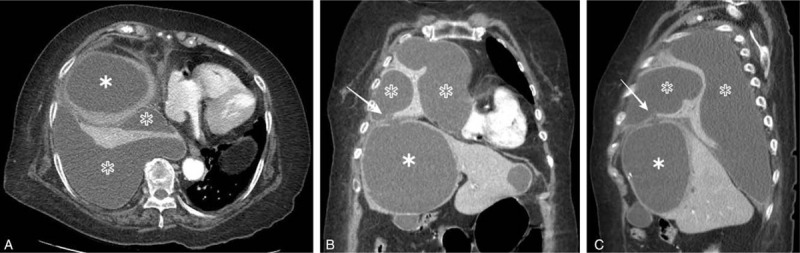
(A): Axial scan (B): Coronal scan (C): Sagittal scan. The initial axial CT scan (A) showed a large abscess (asterisk) that occupied most of the right lobe of the liver at hepatic S4 region which extended into the right pleural space. Coronal, sagittal reformatted images (B, C) from the CT data showed the hepatopleural fistula (arrow). There was a large amount of right empyema (empty asterisk) with diffuse pleural thickening, passive atelectasis of the right lung, and mediastinal shifting to left.
